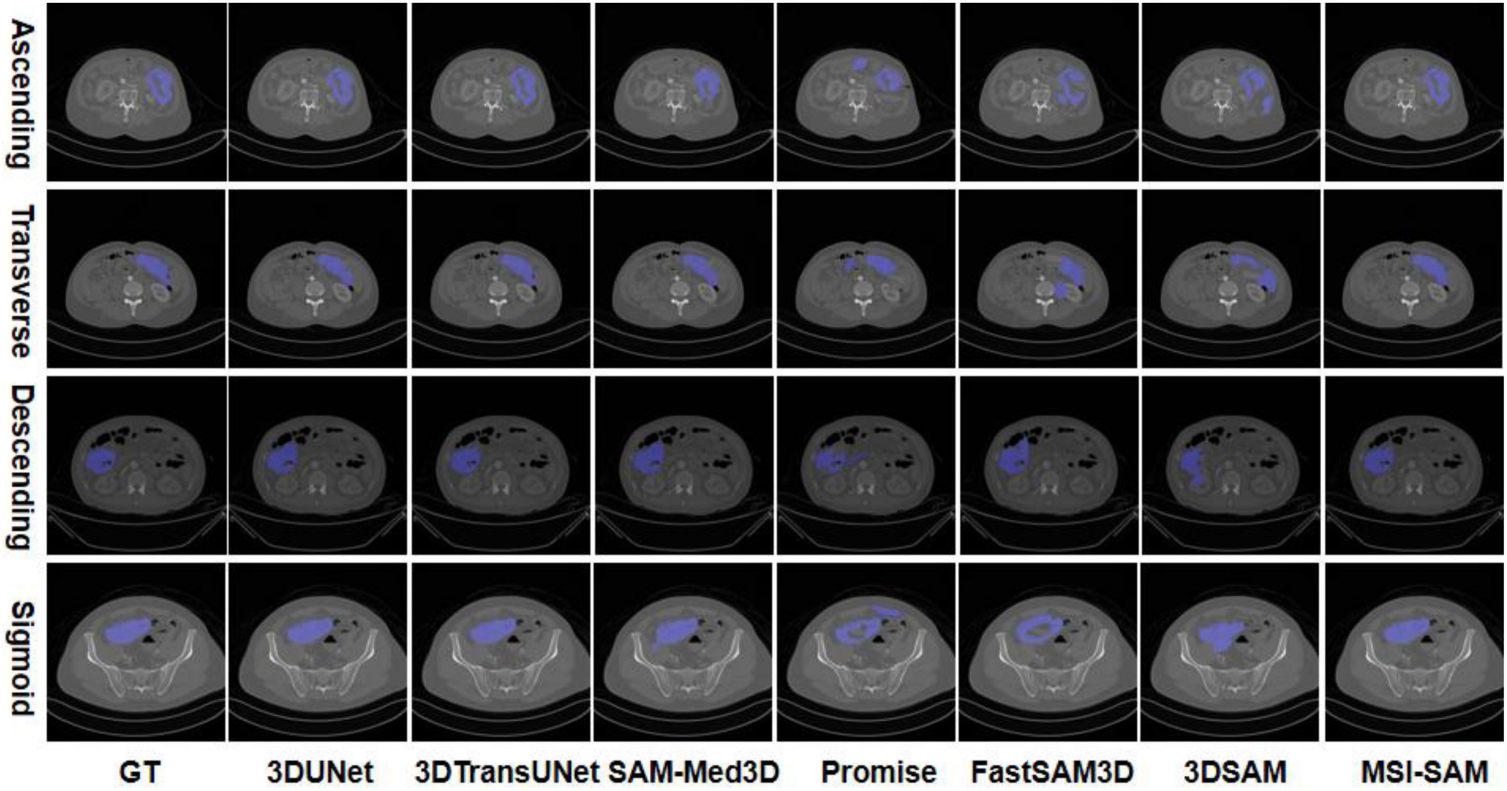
Qualitative visualization of the proposed method, MSI-SAM with benchmark methods on CT of four colon cancer sites: ascending colon, transverse colon, descending colon, and sigmoid colon. The benchmark approaches include 3DUNet (23), 3DTransUNet (24), SAM-Med3D (25), Promise (26), FastSAM3D (27), and 3DSAM (28).
